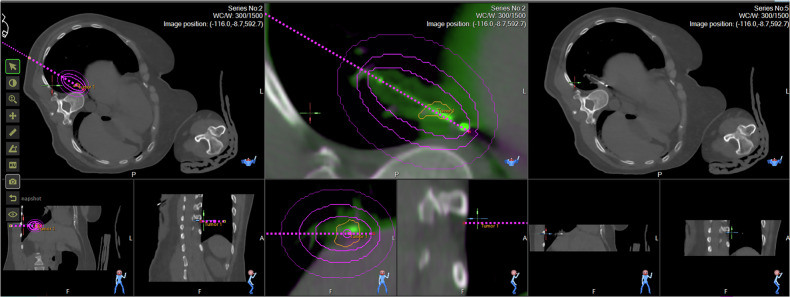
Single-cryoprobe plan and targeting accuracy. Robotic planning and verification display for a 76-year-old man undergoing cryoablation of a subpleural right lower-lobe colorectal metastasis. Left: Planning CT showing the intended cryoprobe trajectory with manufacturer-supplied isotherms. Centre: Fused overlay of planning and verification CT demonstrating close alignment between planned (magenta) and actual (green) needle paths. Right: Verification CT confirming accurate single-probe placement
